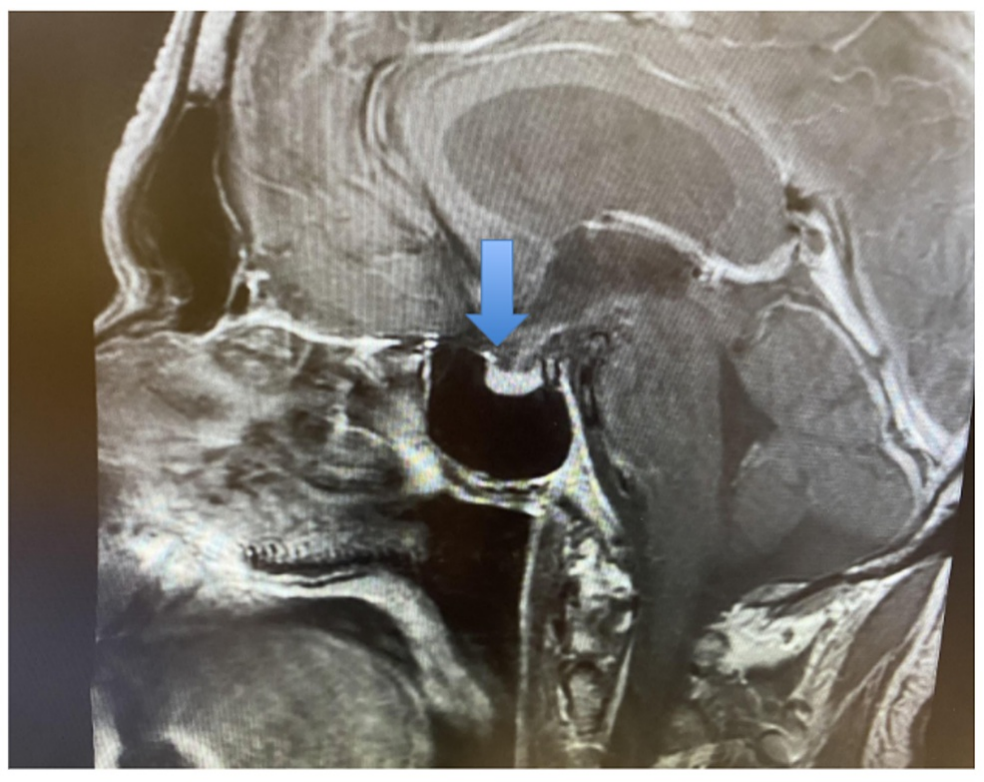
Magnetic resonance imaging (MRI) of the sella pituitary (blue arrow) with and without contrast was obtained, which revealed normal signal and size of the pituitary gland and infundibulum, and no abnormalities of the hypothalamus.
